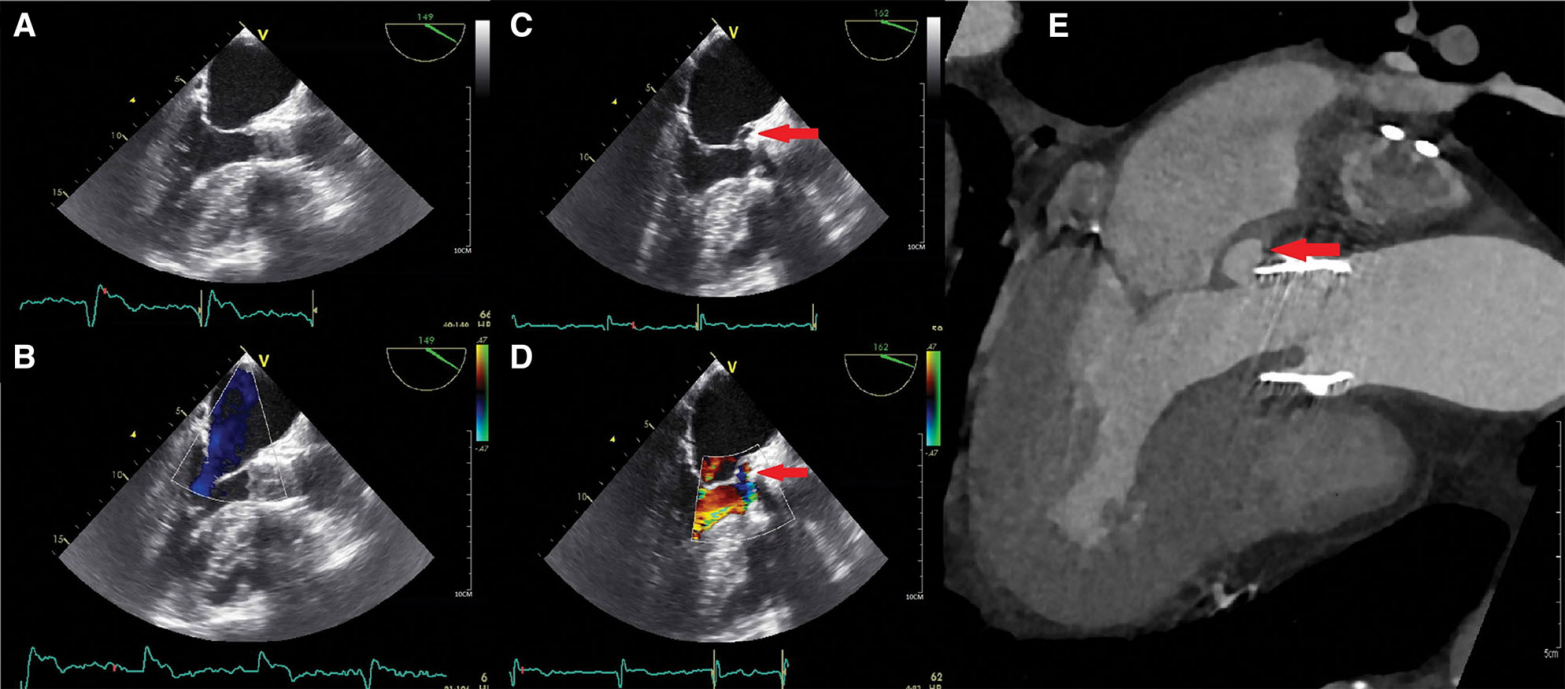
CTA images of a 77-year-old male with suspected TAVI-IE. Initial TEE (A, B) showed only thickened aortic valve leaflets as signs of vegetation. Repeating TEE after a few days (C, D) showed a new aortic regurgitation and a paravalvular space as sign of possible mycotic aneurysm, which was confirmed on the CTA (E)
